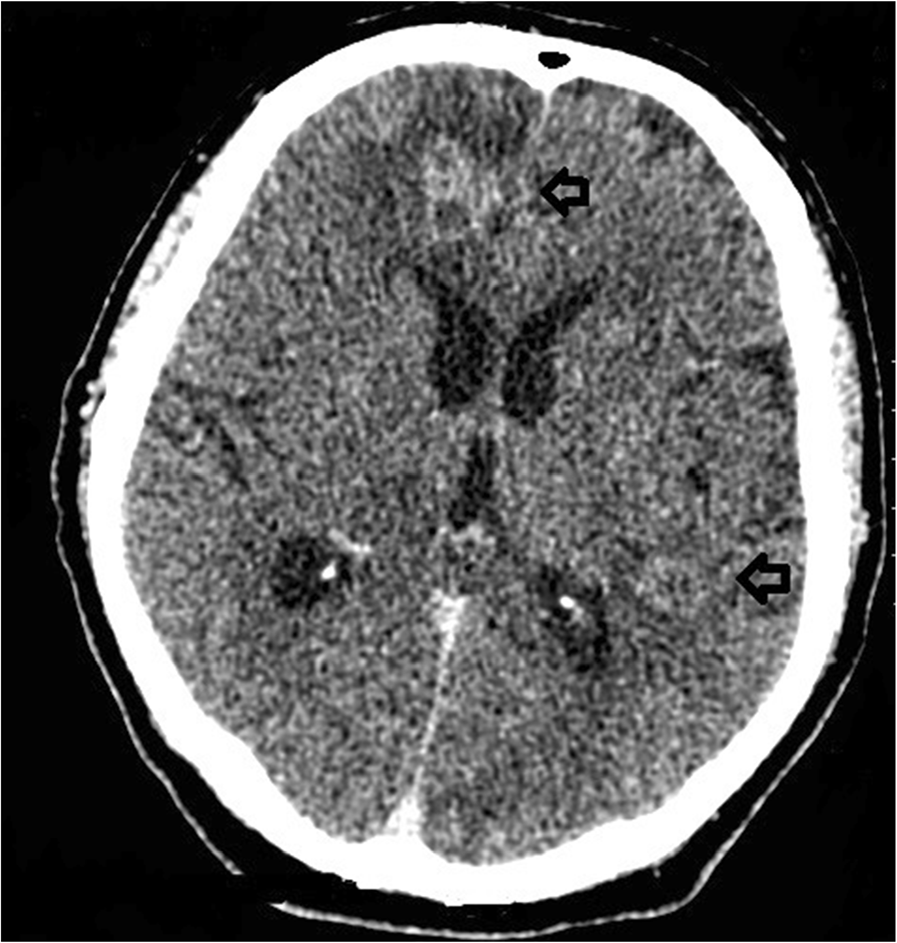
Computed Tomography head with contrast. Computed Tomography head with contrast showing multiple ring enhancing lesions with surrounding oedema (see arrows)
